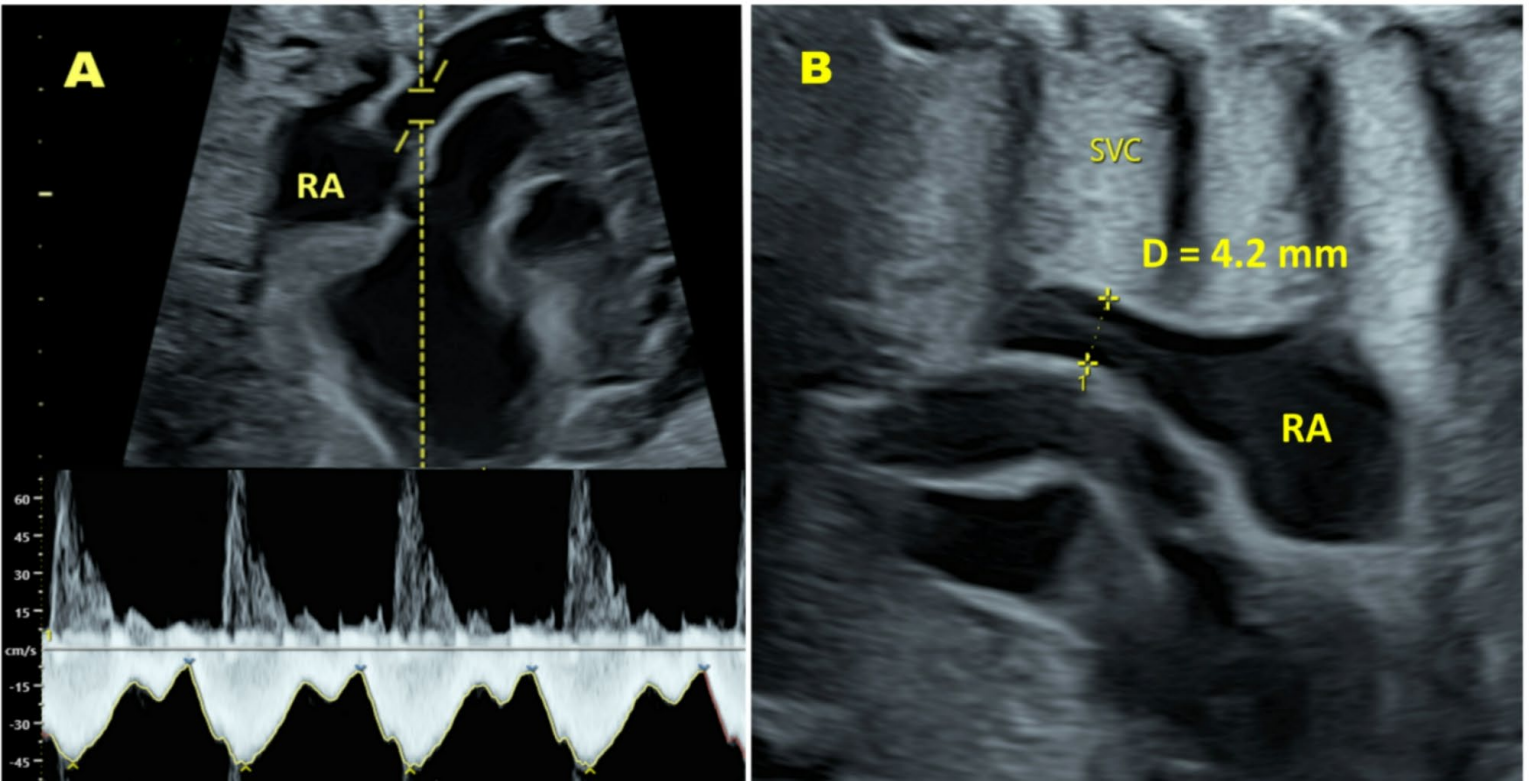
Ultrasound image demonstrating the technique of superior vena cava (SVC) blood flow velocities (A) and diameter (B) measurements. RA = Right atrium. D = SVC diameter. Maximum velocity envelope of the SVC Doppler velocity waveforms (below the baseline) is traced. Doppler velocity waveforms above the baseline represent the ascending aorta velocities.
